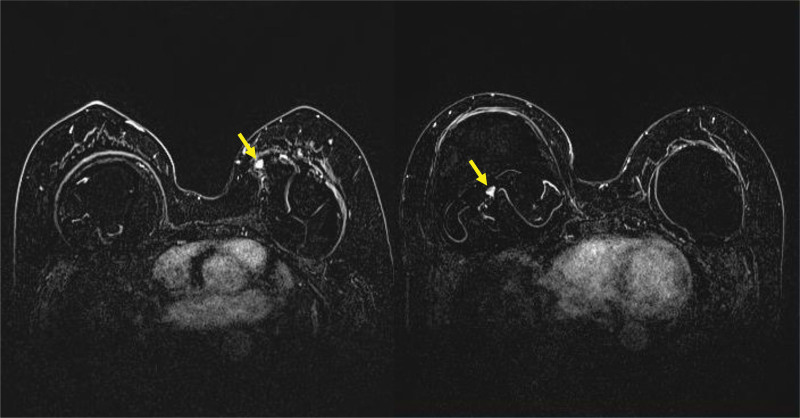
Breast magnetic resonance imaging (MRI). On MRI, fluid collection along with implant rupture on both sides was suspected. Several enhancing nodules (yellow arrows) were identified.
